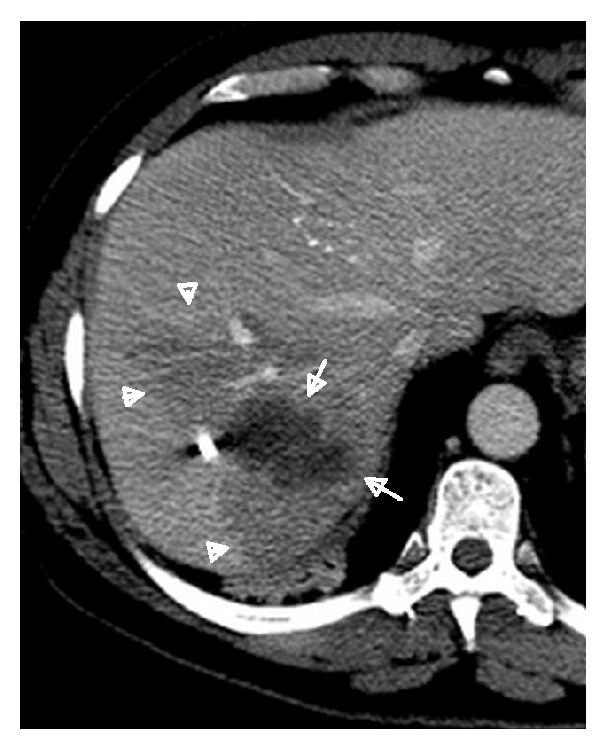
49-year-old female patient with melanoma. In the CT scan performed 3 months after treatment a hypodensity (arrowheads) around the treated lesion (arrows) is seen. Of note, a radiopaque fiducial seed is seen laterally to the lesion.
